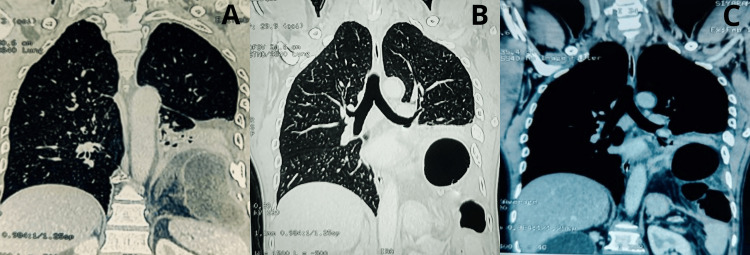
CT of the thorax, along with a defect in the left side (posterior aspect) of the diaphragm along with the abdominal contents A-C: Left-side traumatic diaphragmatic hernia in Cases 1, 2, and 3, respectively
